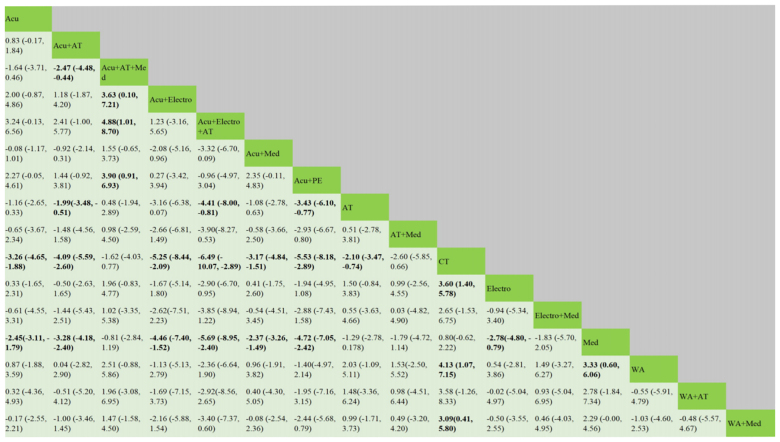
Network meta-analysis results for PSQI total scores. The bold font indicates a statistical difference. Acu = acupuncture, AT = auriculotherapy, CT = conventional treatment, Electro = electroacupuncture, Med = western medicine, PE = point embedding, PSQI = Pittsburgh Sleep Quality Index, WA = warm acupuncture.
